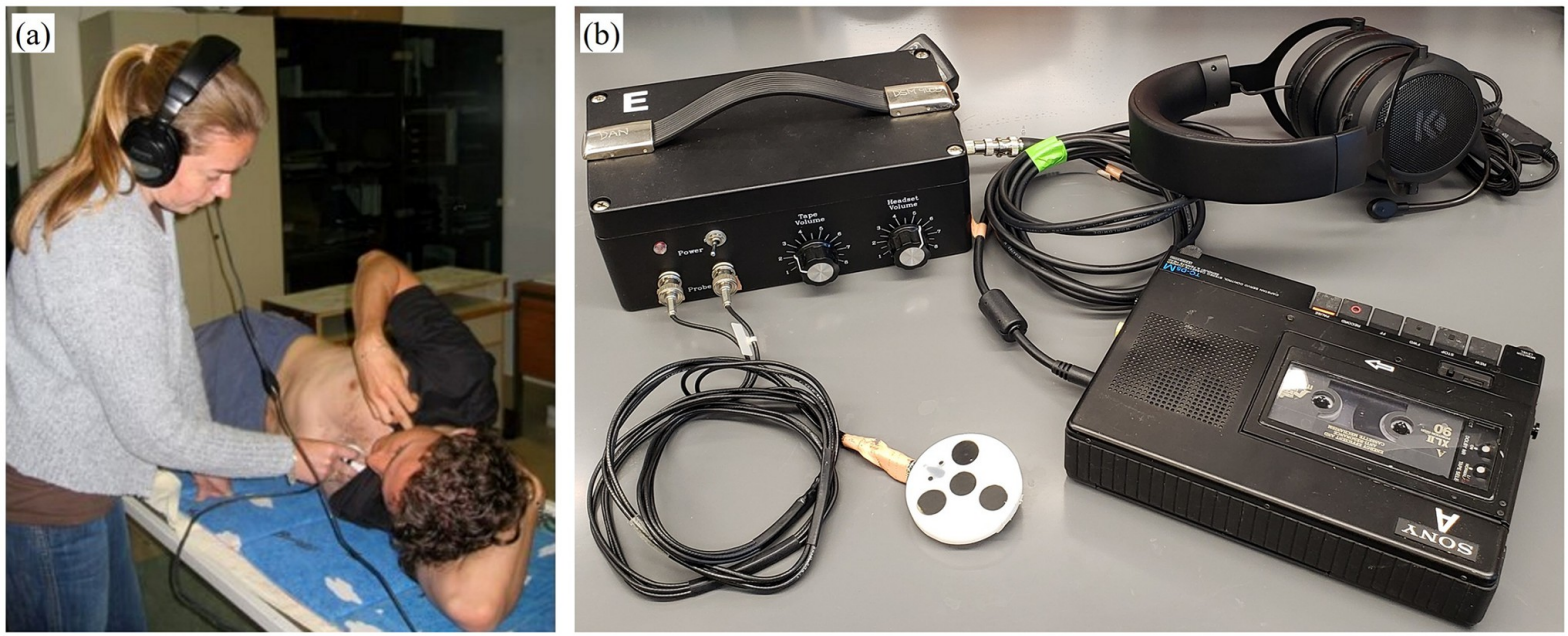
Doppler ultrasound measurement: (a) Doppler technician making a precordial measurement; (b) a portable Doppler ultrasound device (Doppler bubble monitor DBM9008; Techno Scientific Inc., Concord, Canada) with probe and tape recorder for detection. Bubbles are most typically measured over the subclavian vein and the precordial region.
